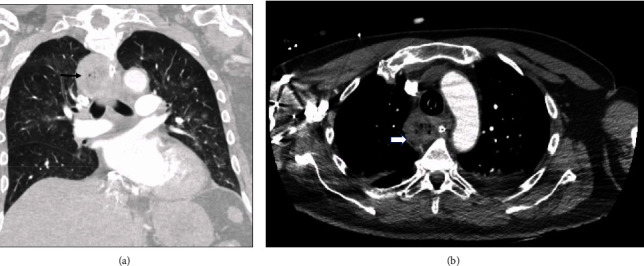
(a) Coronal view of CT chest with contrast showing a large impacted food bolus just above the carina (black arrow). (b) Axial view of CT chest with contrast showing food bolus with air (white arrow). A nasogastric tube can be seen traversing the food bolus.
